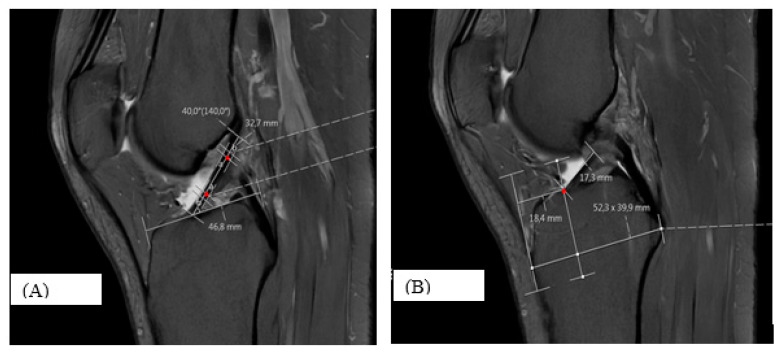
Sagittal MRI measurements of the ACL tibial insertion.
